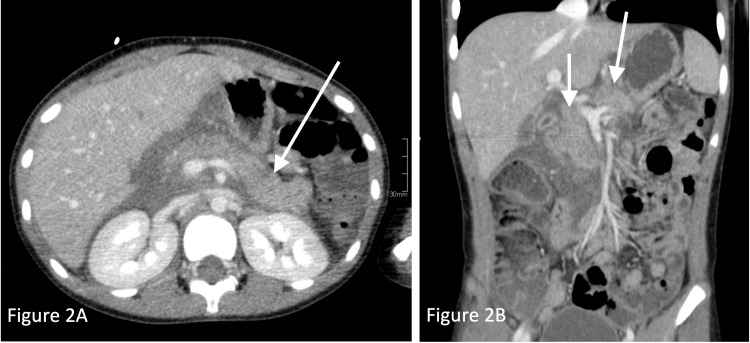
CT abdomen and pelvis with IV contrast revealing pancreatic enlargement, peripancreatic fluid, and intraperitoneal and retroperitoneal fluid, raising concerns for acute pancreatitis.
